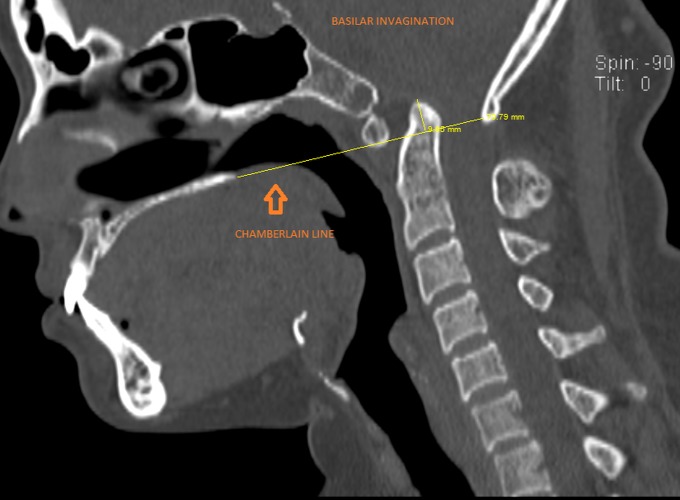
Sagittal computed tomogram showing basilar invagination (tip of the odontoid is located above the Chamberlain line)
